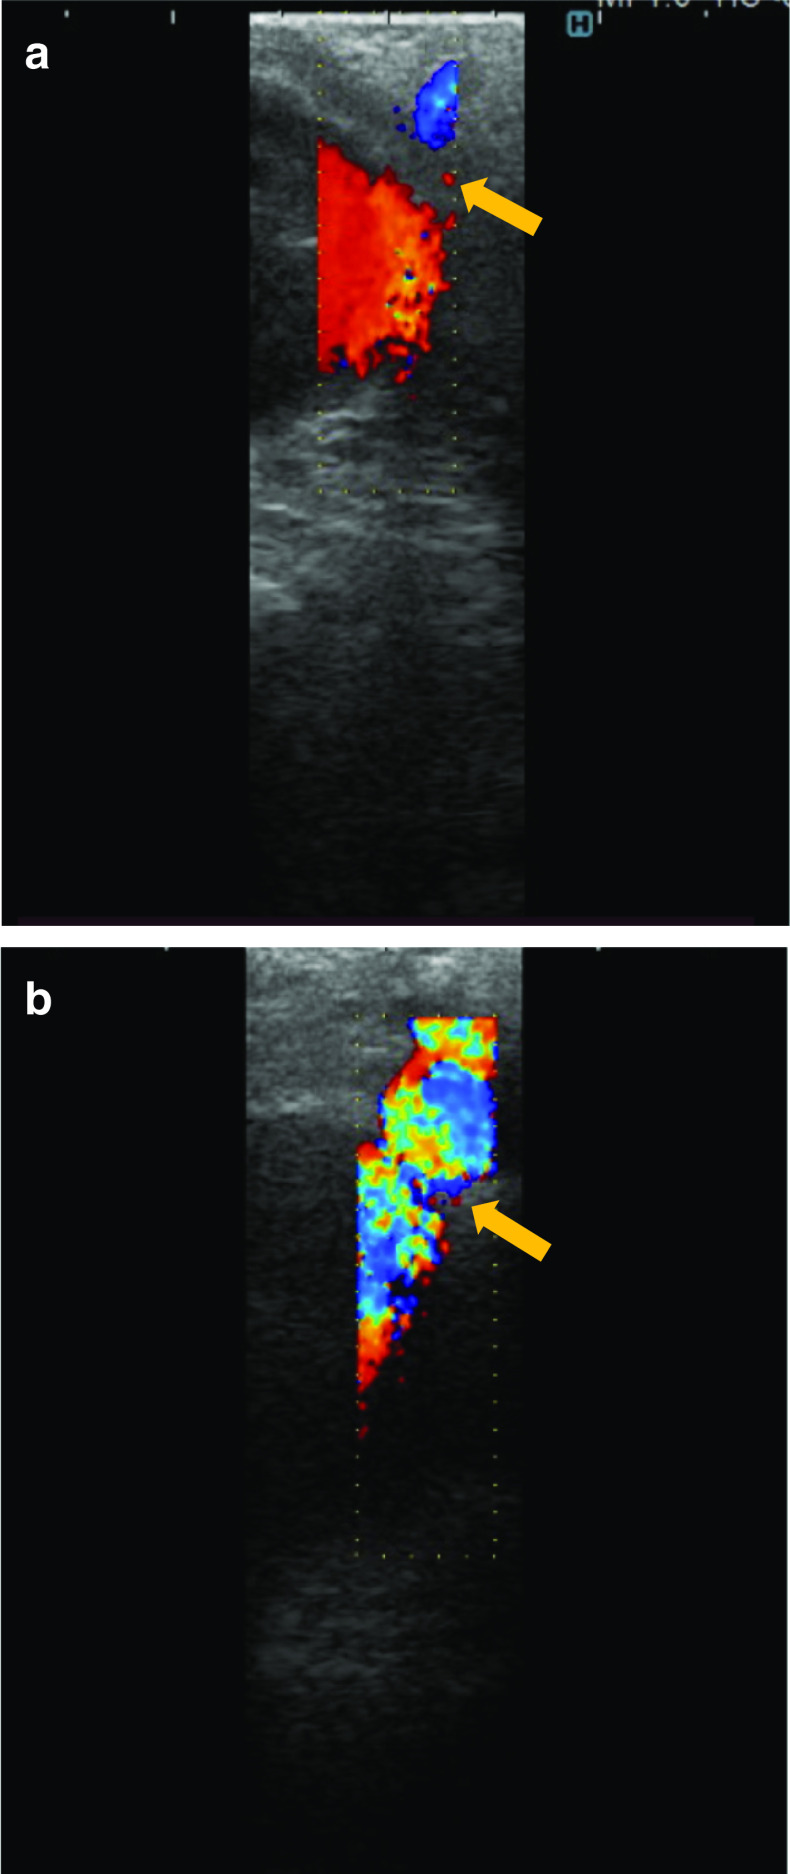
Laparoscopic ultrasonography. a Stenosis of the celiac artery origin was confirmed. b After MAL resection, release of the stenosis was confirmed
